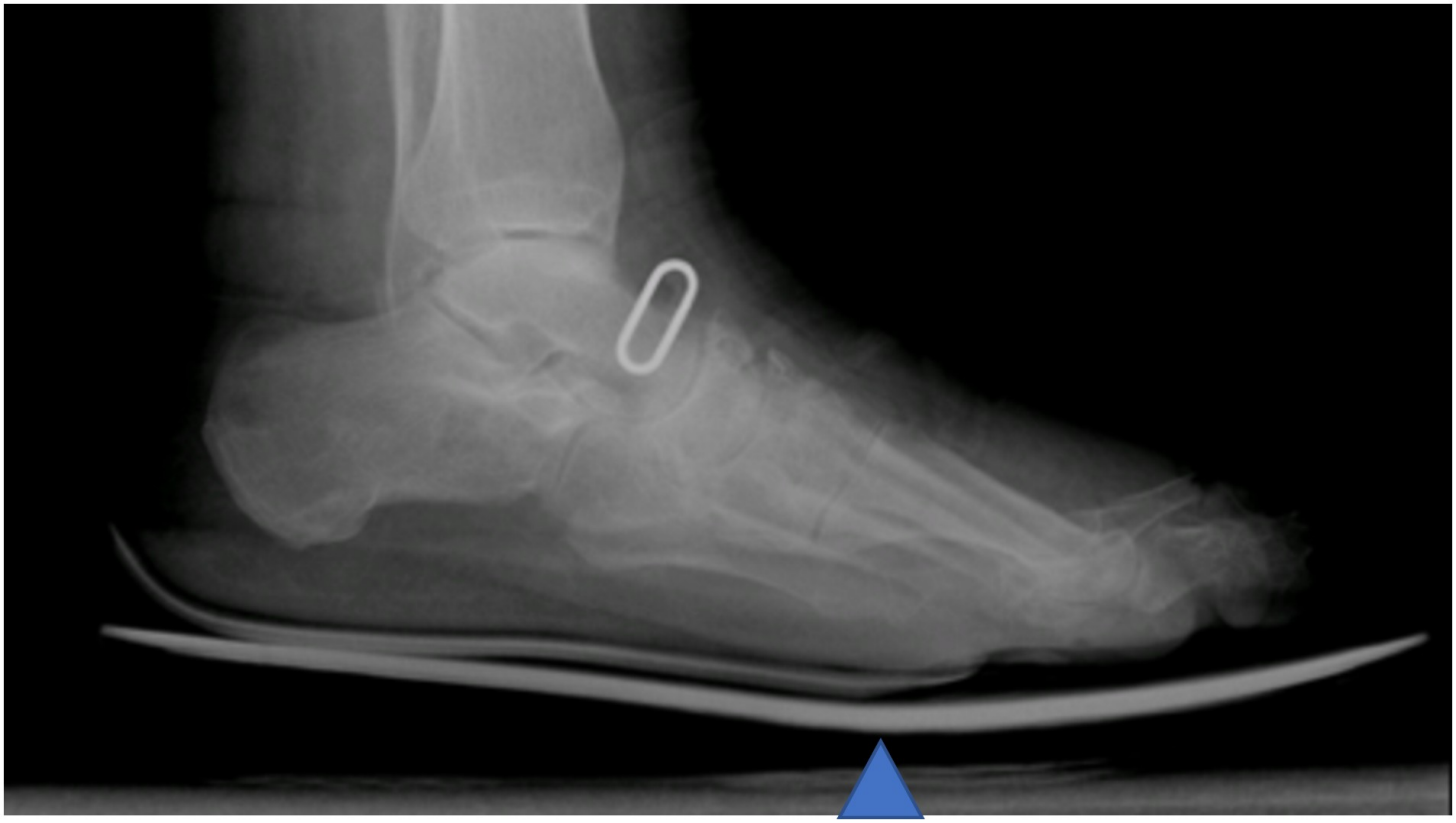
Weight-bearing lateral X-ray of the shod feet.
